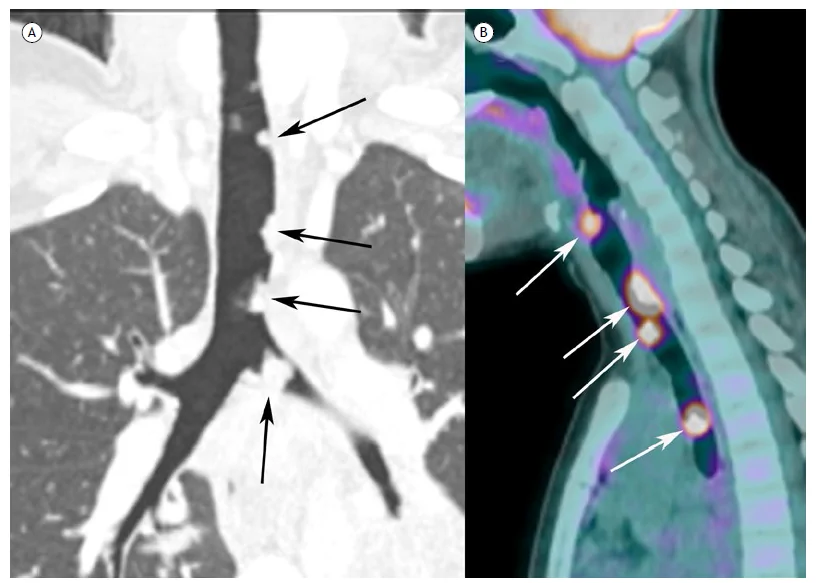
In A, Coronal CT of the thorax showing multiple nodular lesions in the trachea and bronchial walls (arrows). In B, 3D sagittal reconstruction of an 18F-FDG PET/CT image showing lesions in the trachea and bronchi with high glycolytic activity (arrows).
